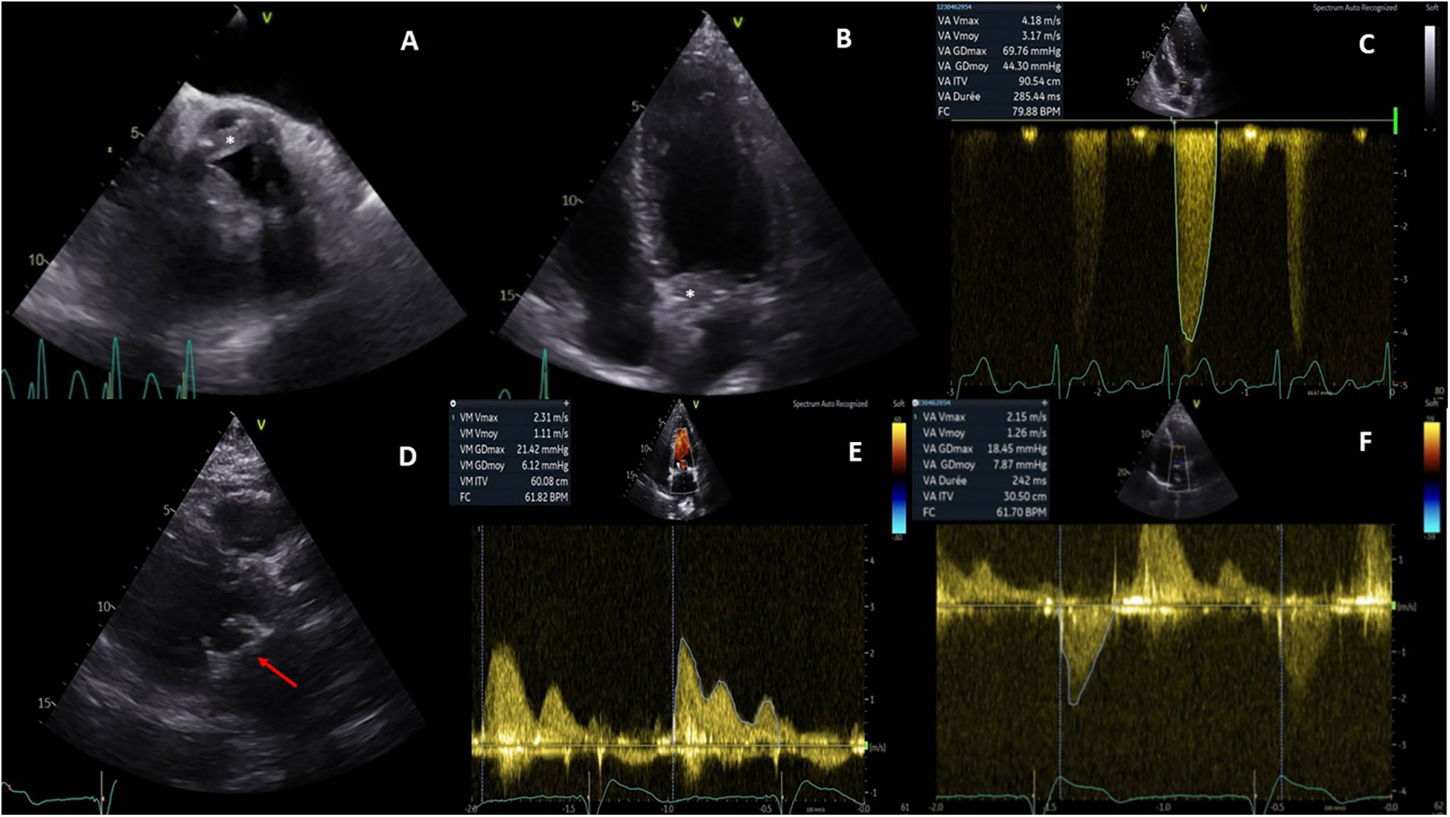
Pre- and postoperative echocardiography. Preoperative echocardiography showed an abnormal thickening of aortic cusps (*, A) extending to the anterior leaflet of the mitral valve (*, B) and resulting a significant subaortic obstruction (maximal gradient of 70 mmHg, C). Postoperative echocardiography demonstrated a well-positioned mitral bioprosthesis (arrow, D); the mean transprosthetic gradient was 1.1 mmHg (E) in the mitral position and 7.8 mmHg (F) in the aortic position.
